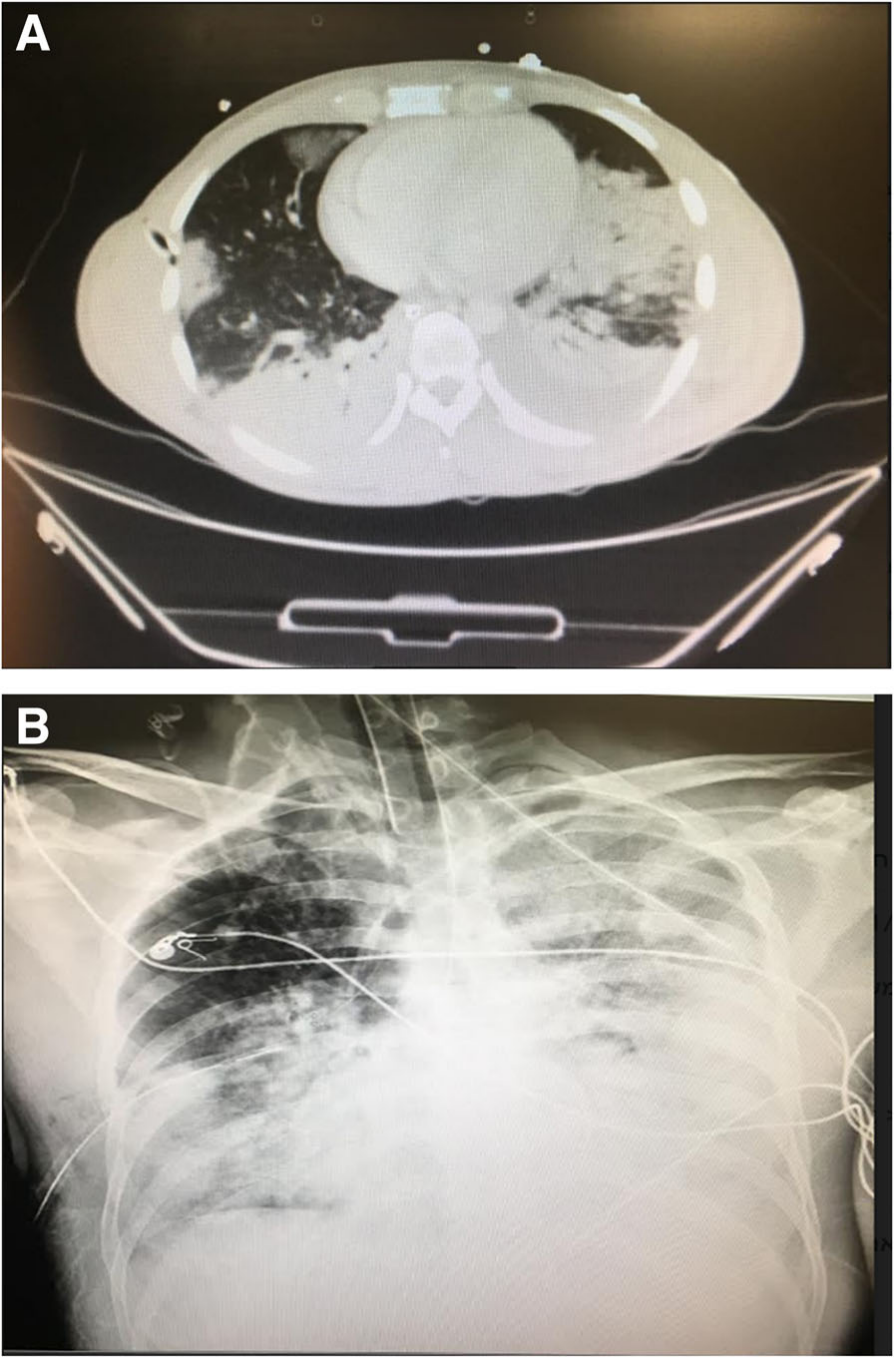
a Computed tomogram of the patient with ARDS, demonstrating bilateral dependent consolidation, a large consolidation in the LUL, and small peripheral patchy alveolar infiltrate on the right. b A chest radiograph of the patient on the 6th day of hospitalization, showing ARDS with bilateral infiltrates and bilateral heterogeneous consolidations, more on the left side
